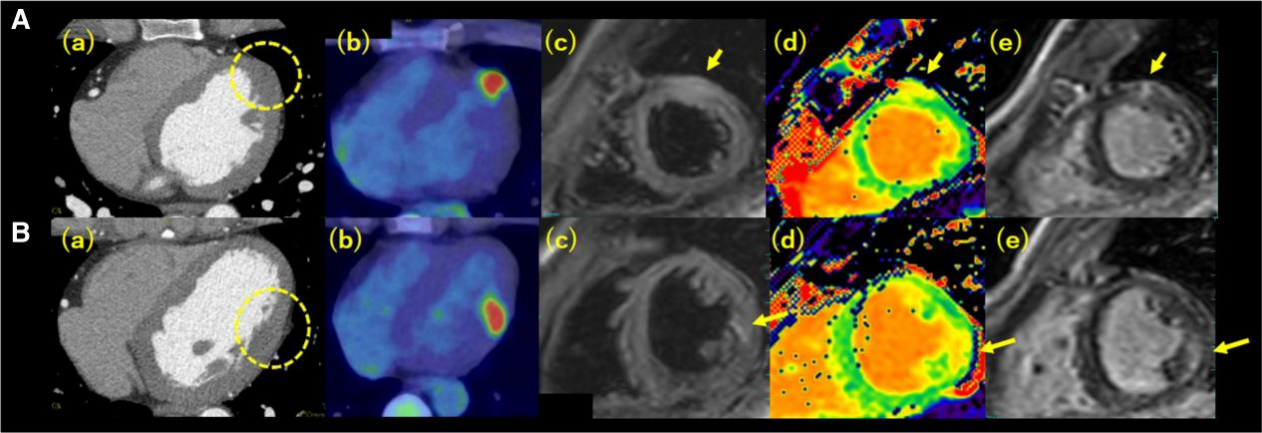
(*A*) A mass is in the middle of the left ventricular anterolateral wall (arrows and circle). (*B*) The other mass is located between the papillary muscles of the left ventricular posterolateral wall (arrows and circle). (*a*) Axial of computed tomography (CT) shows areas of pale low CT values. (*b*) Positron emission tomography CT (PET-CT) axial shows fluorodeoxyglucose (FDG) accumulation. (*c*) Delayed contrast sagittal of contrast magnetic resonance imaging (MRI) shows high signal. (*d*) T1 mapping sagittal of contrast MRI shows a different image than normal myocardium. (*e*) T2 emphasis sagittal of contrast MRI shows mildly high signal.
